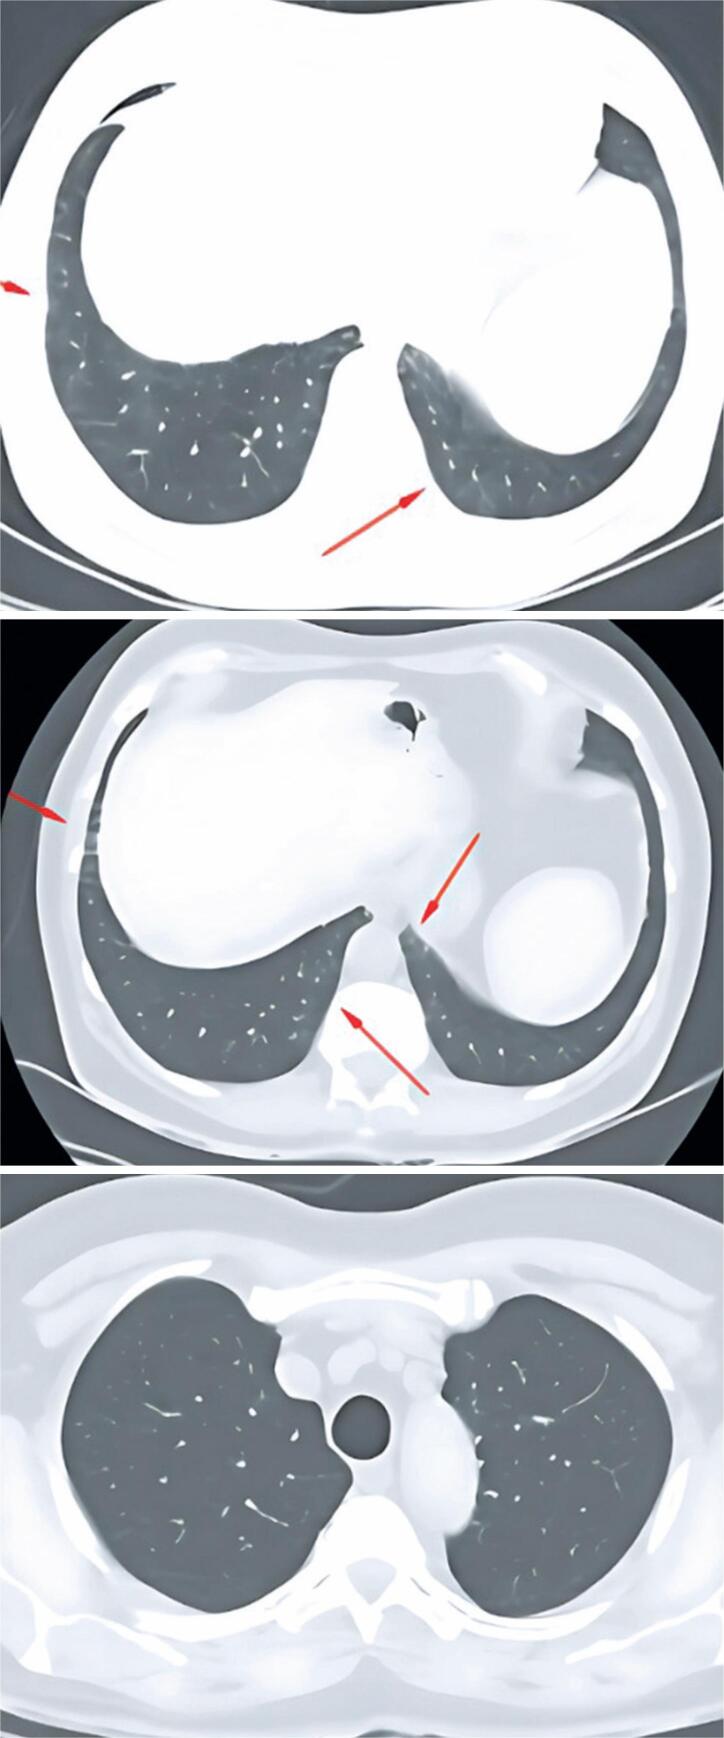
Chest CT in axial plane. Arrows indicate subtle ground-glass and reticular opacities
